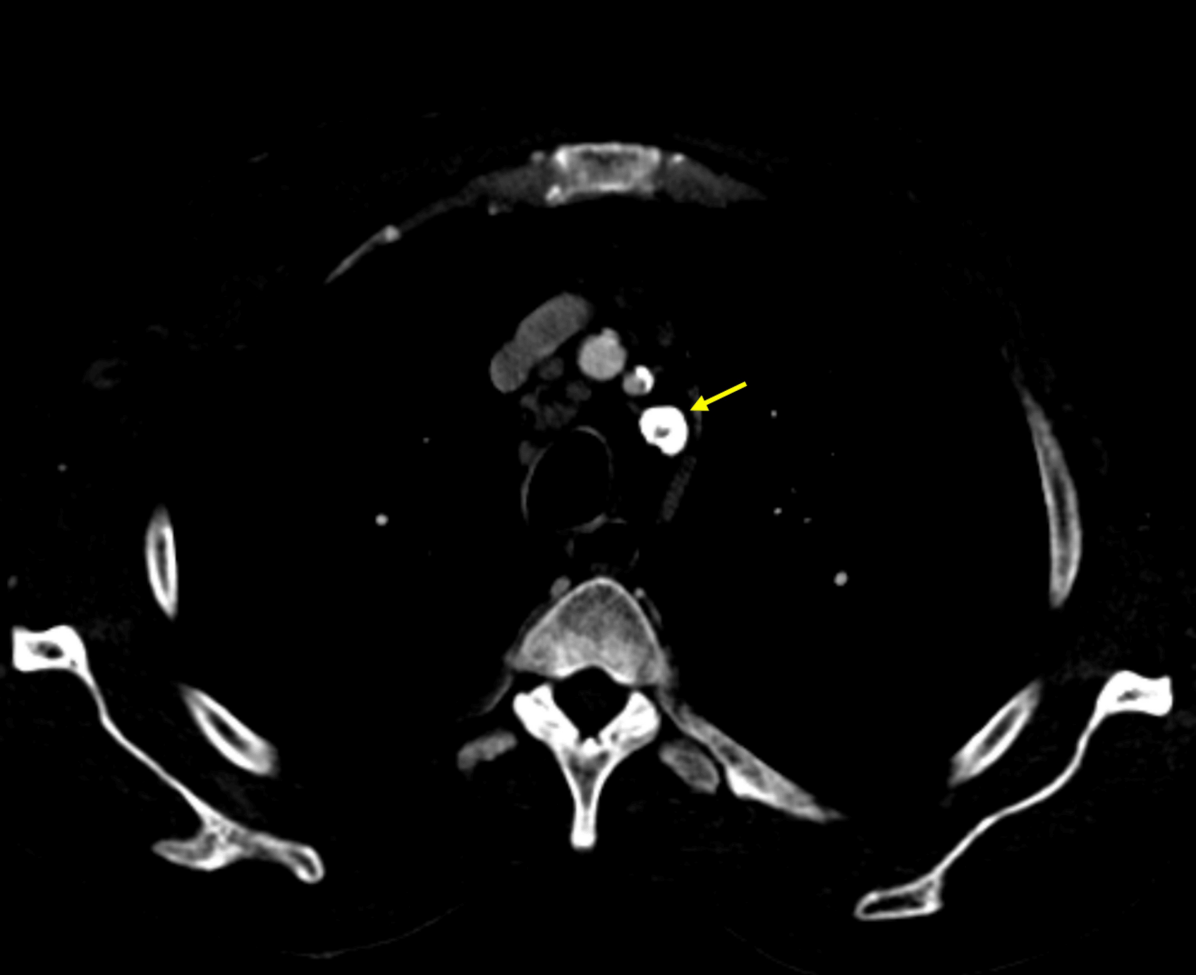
Axial CT scan of the left brachiocephalic trunk origin (yellow arrow)
